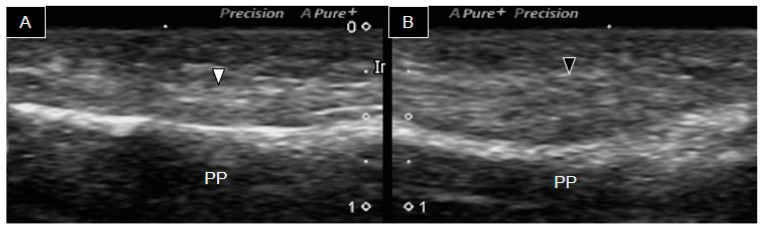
Ultrasound image of the palmar side of the third right (A) and left (B) fingers. The white arrowhead indicates the right finger flexor tendon, whereas the black arrowhead indicates the left flexor tendon. PP, proximal phalanx.
